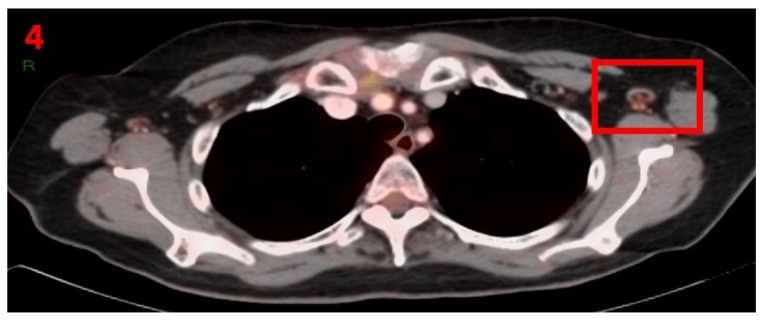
[18F]FDG PET-CT showing hypermetabolic uptake in the 4th patient with lymphadenopathy in the left axillary region (red square).
